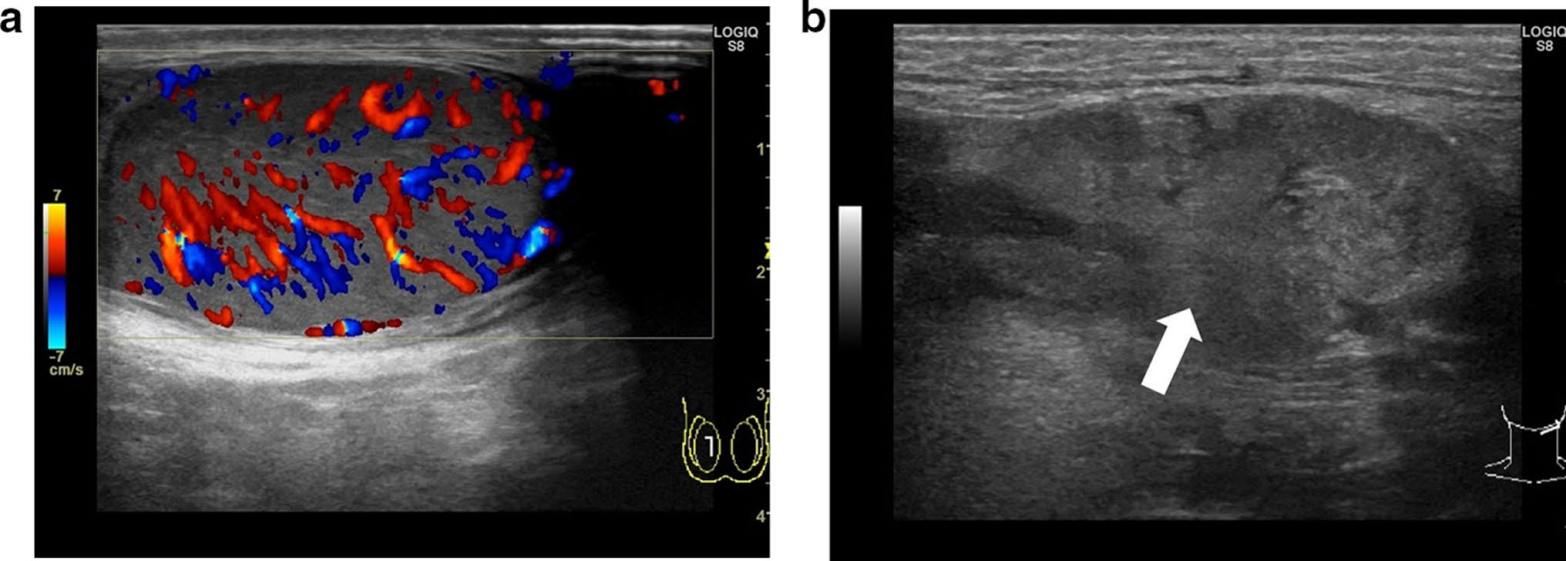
Ultrasonographic images of a 11-month-old boy with orchitis due to mumps infection. a The right testis is enlarged. Color Doppler sonogram shows hypervascularity within the testis. b The submandibular gland is swollen. Orchitis due to mumps infection was diagnosed based on these findings
